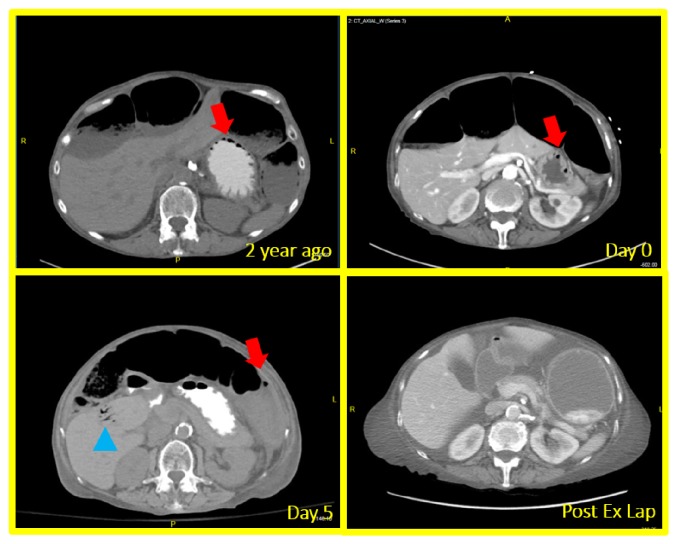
CT of abdomen revealed chronic bowel dilation and pneumatosis intestinalis ((a) and (b)); emergency of portal vein gas and worsening pneumatosis intestinalis (c); improvement after subtotal colectomy (d). Red arrow: pneumatosis intestinalis; blue arrow: portal vein gas.
